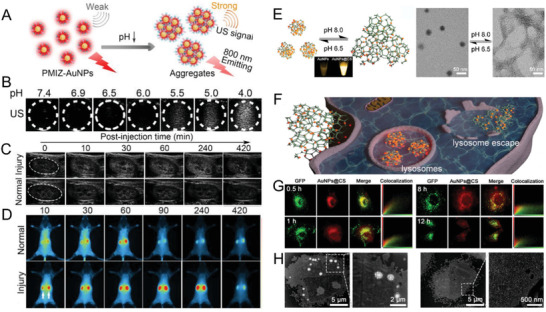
Stimuli‐responsive functional nanoassemblies. A) Scheme of pH‐induced aggregation and ultrasound contrast. B) Ultrasound imaging of PMIZ‐AuNPs at different pH values. Ultrasound imaging C) and fluorescence imaging D) of kidney injury with PMIZ‐AuNPs. Reproduced with permission.^[^
